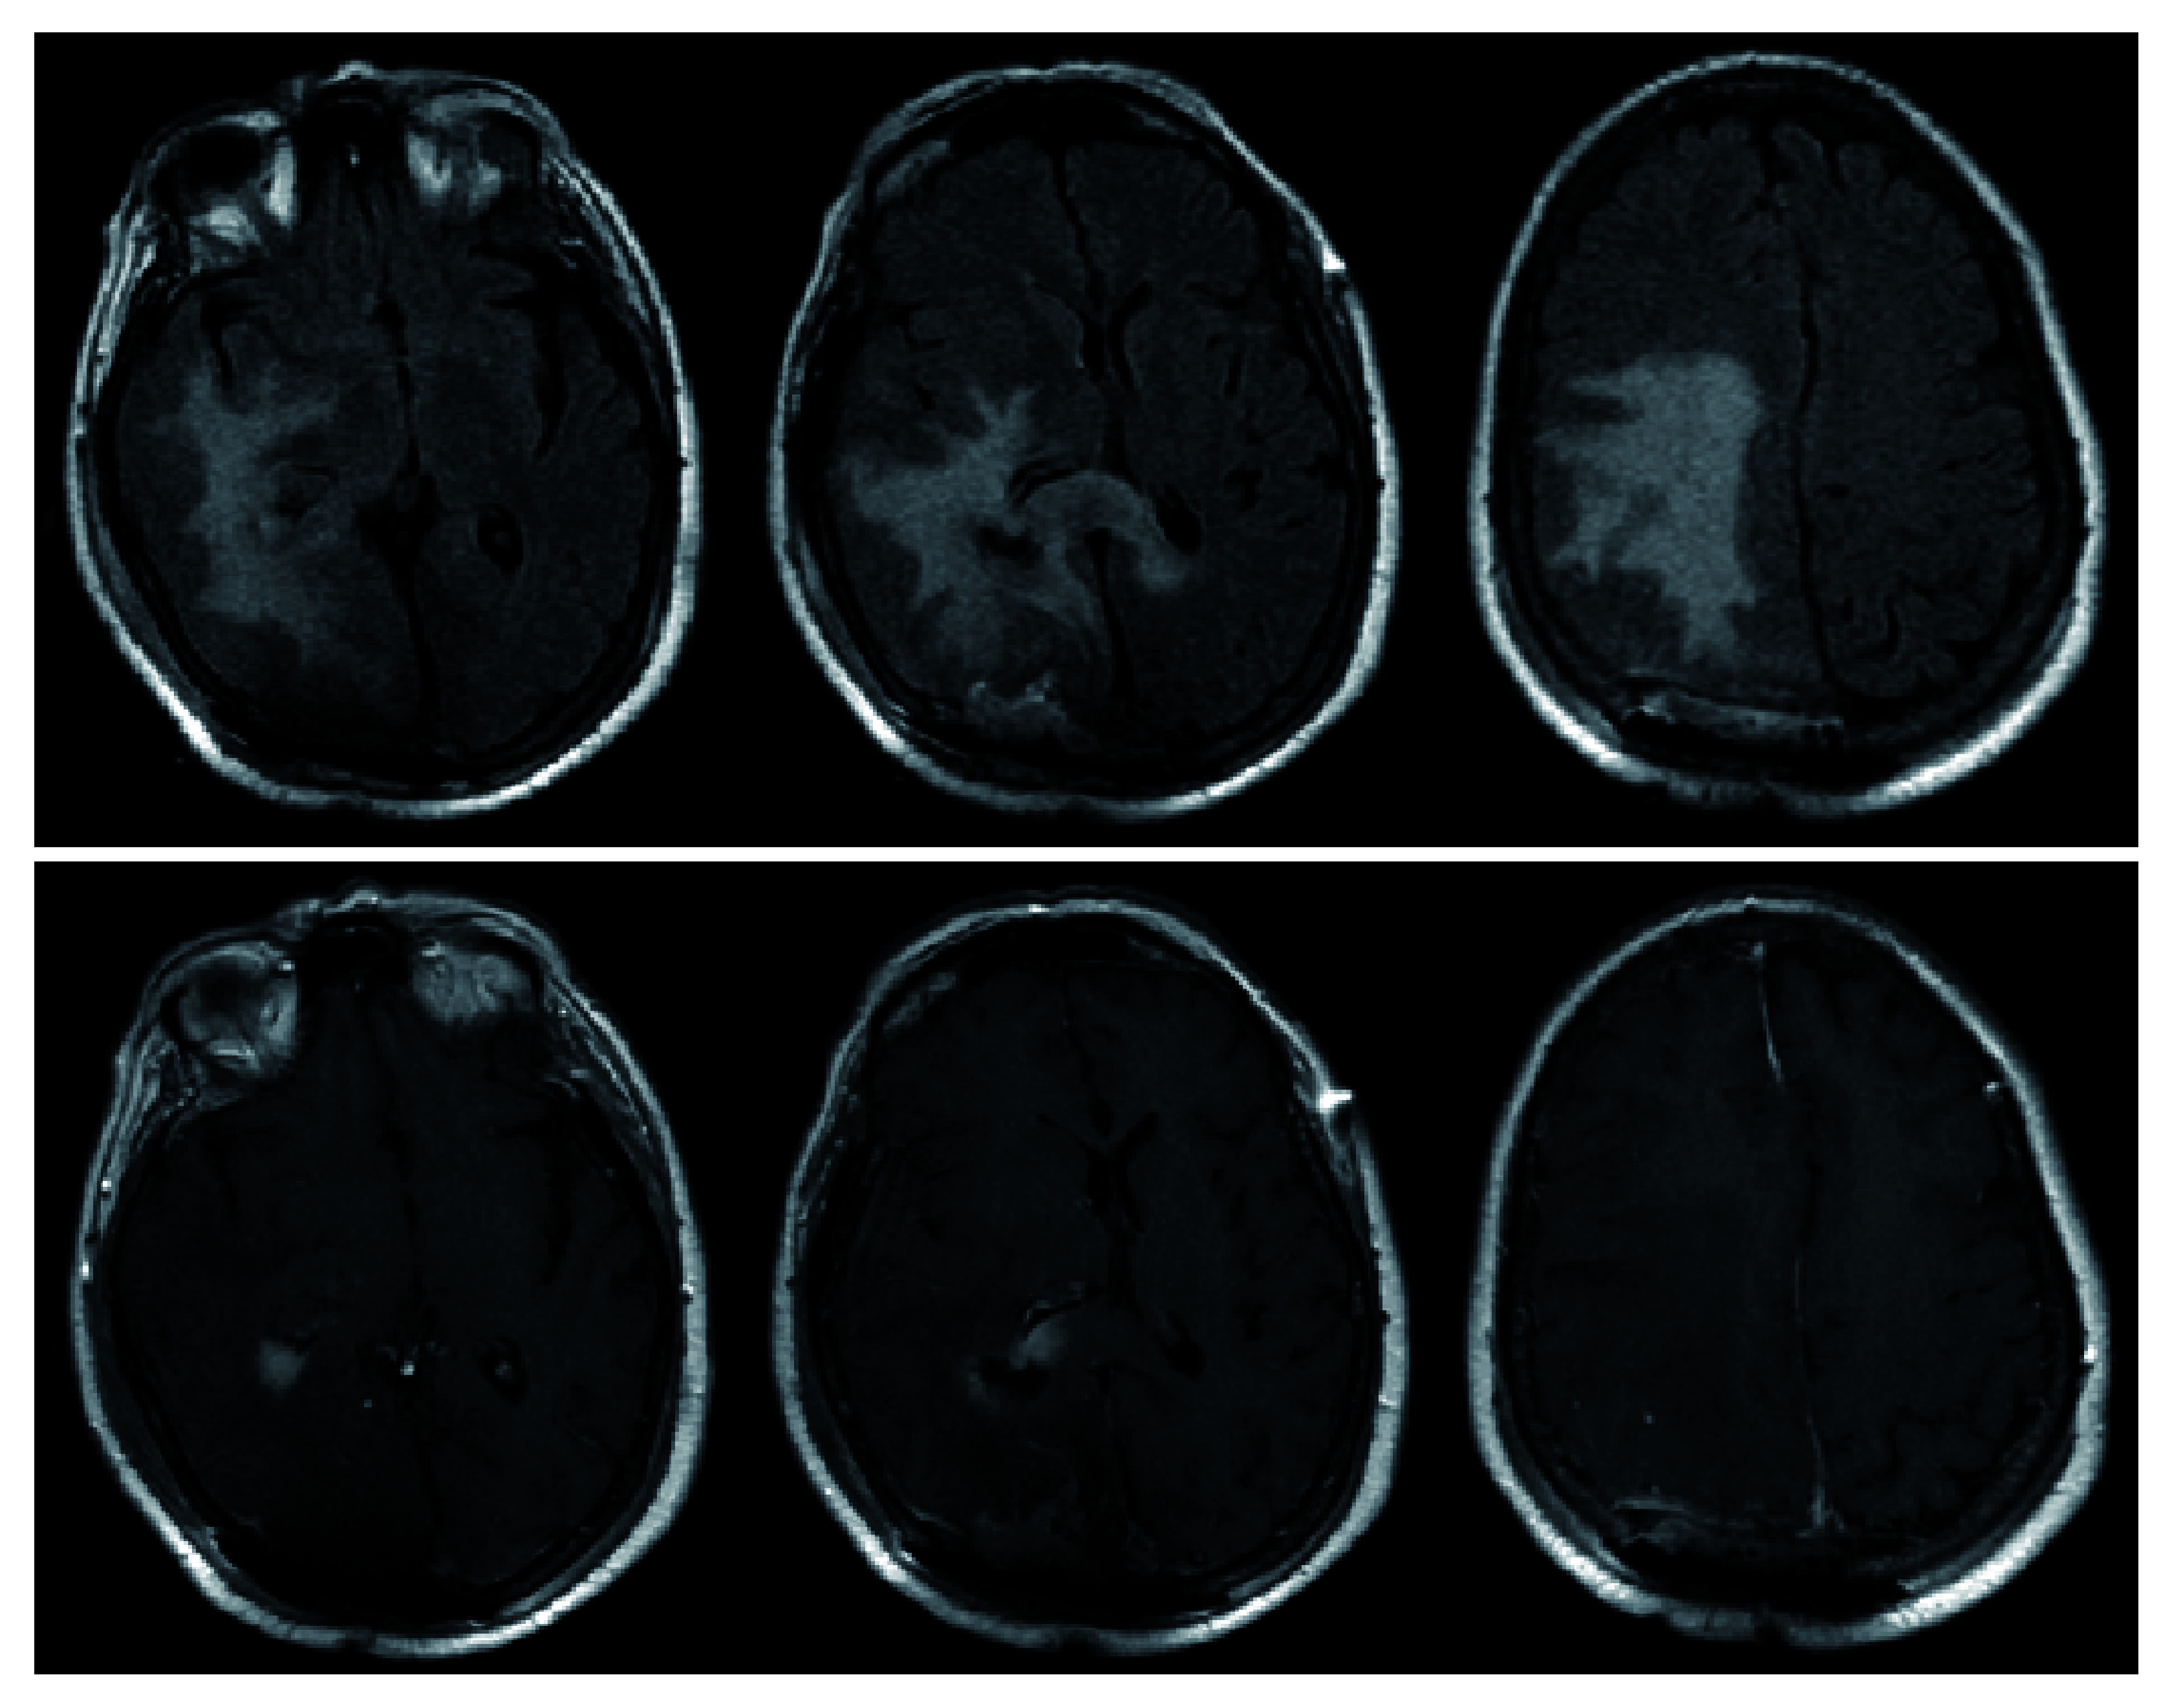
Post-operative MRI demonstrating similar FLAIR changes as pre-operative MRI (top). T1-post contrast reveals gross-total resection of the previously seen extra-axial lesion in the right parieto-occipital region as well as subtotal resection of right periventricular lesion (bottom). The midline shift is significantly improved from pre-operative MRI (
